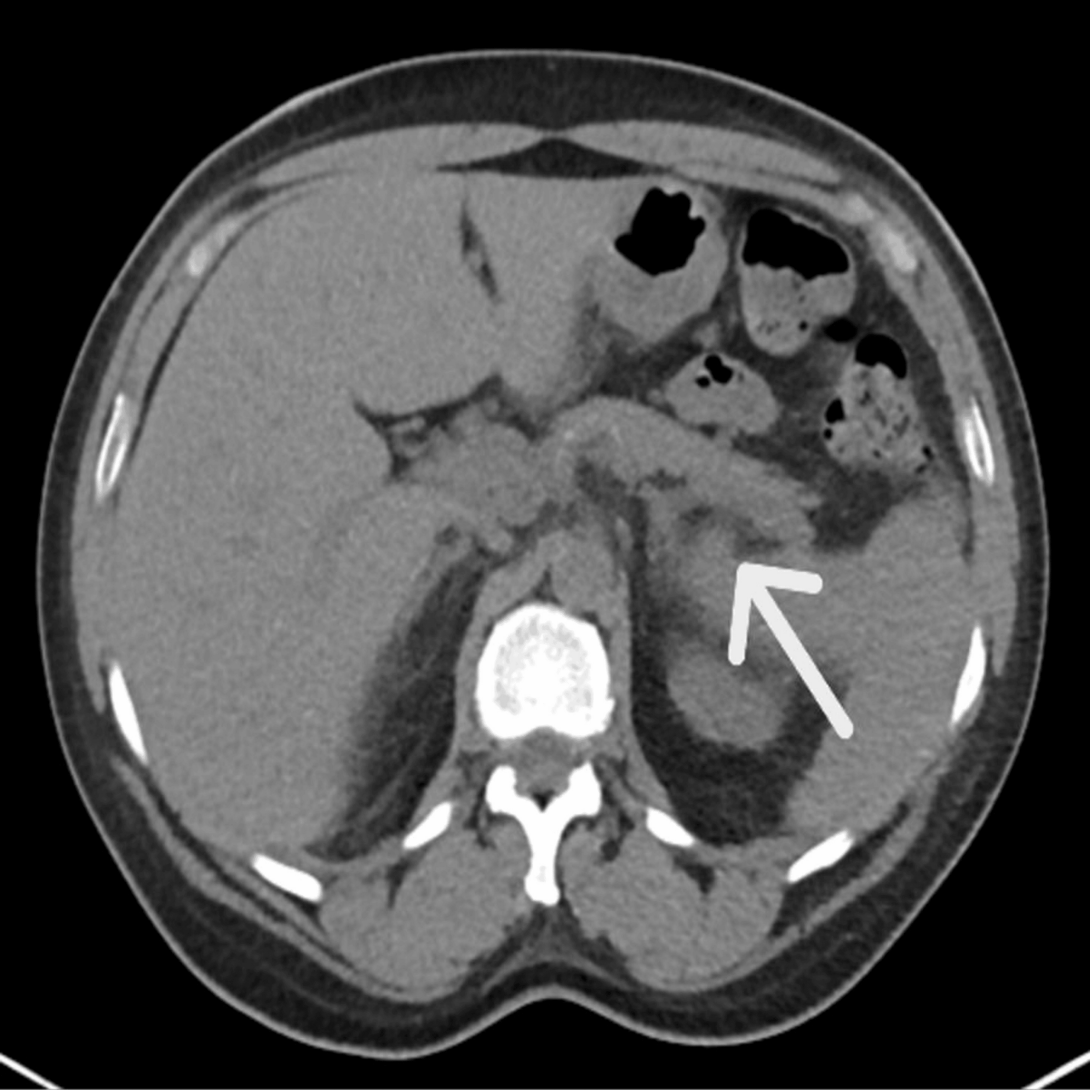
CT without contrast transverse view In this image, the borders of the pancreas are blurry, suggesting pancreatitis (white arrow). Other organs such as the liver and spleen, also evident here, are unremarkable.
